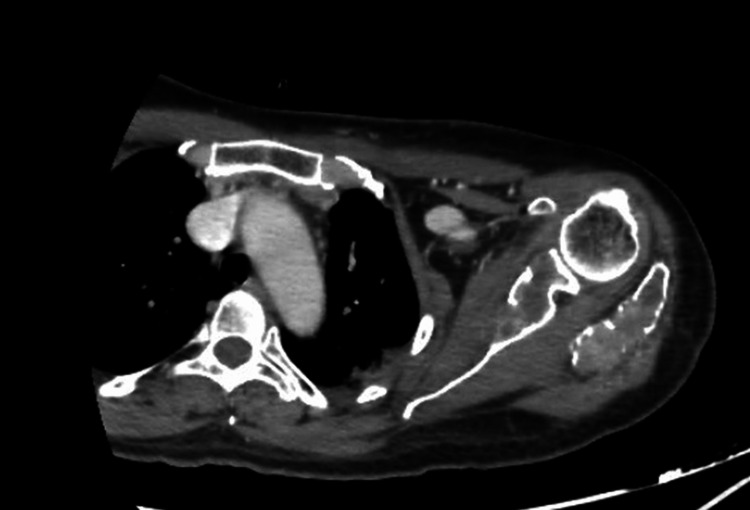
Axial follow-up CT of the shoulder showing decreased surrounding soft-tissue involvement.
